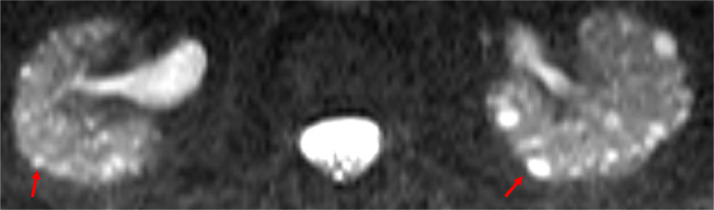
Select axial T2 slice from MRI demonstrates normal-sized kidneys with multiple 1-2 mm sized T2 hyperintense renal cysts (red arrows) located within both the renal medulla and cortex.
